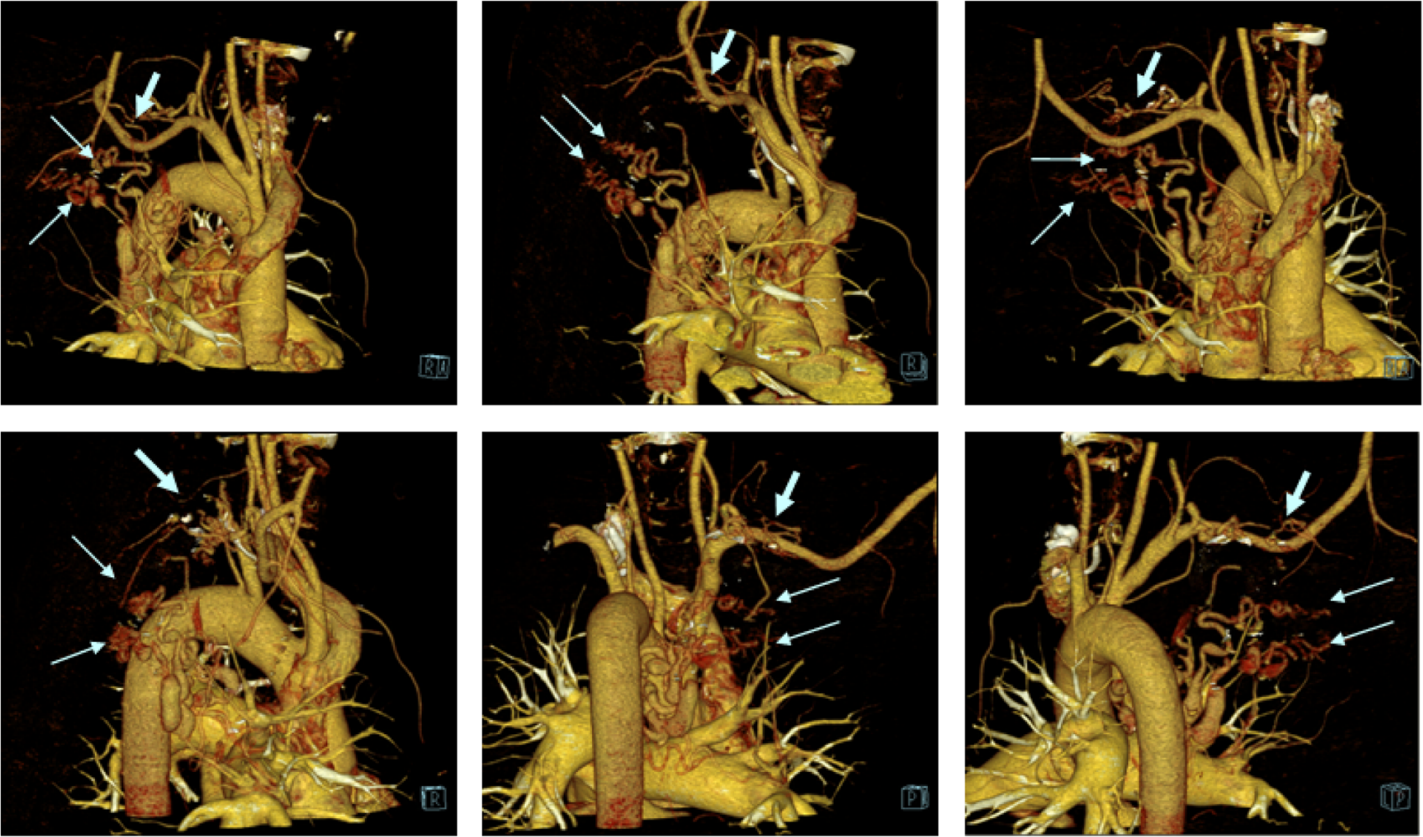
Systemic artery to pulmonary artery fistulas, CT angiography reconstruction; bronchial arteries fistulas (narrower arrows) and subclavian artery fistulas (thicker arrow)
